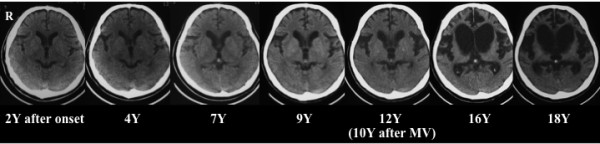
Computed tomography scans of the younger sibling. At the age of 48 years (12 years after onset; 10 years after the initiation of mechanical ventilation) brain atrophy was apparent. Subsequently, brain atrophy progressed as in his elder sister. Y-Years; MV-mechanical ventilation.
